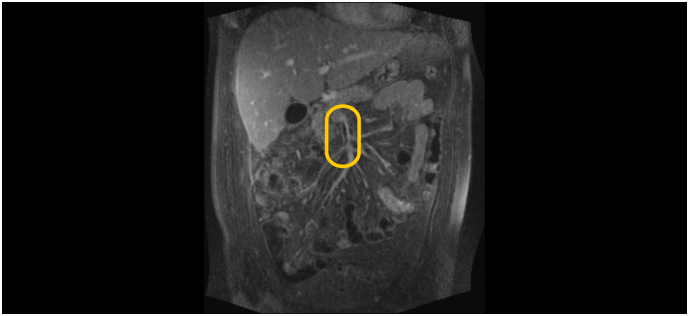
MRI scan of portal vein thrombosis.
